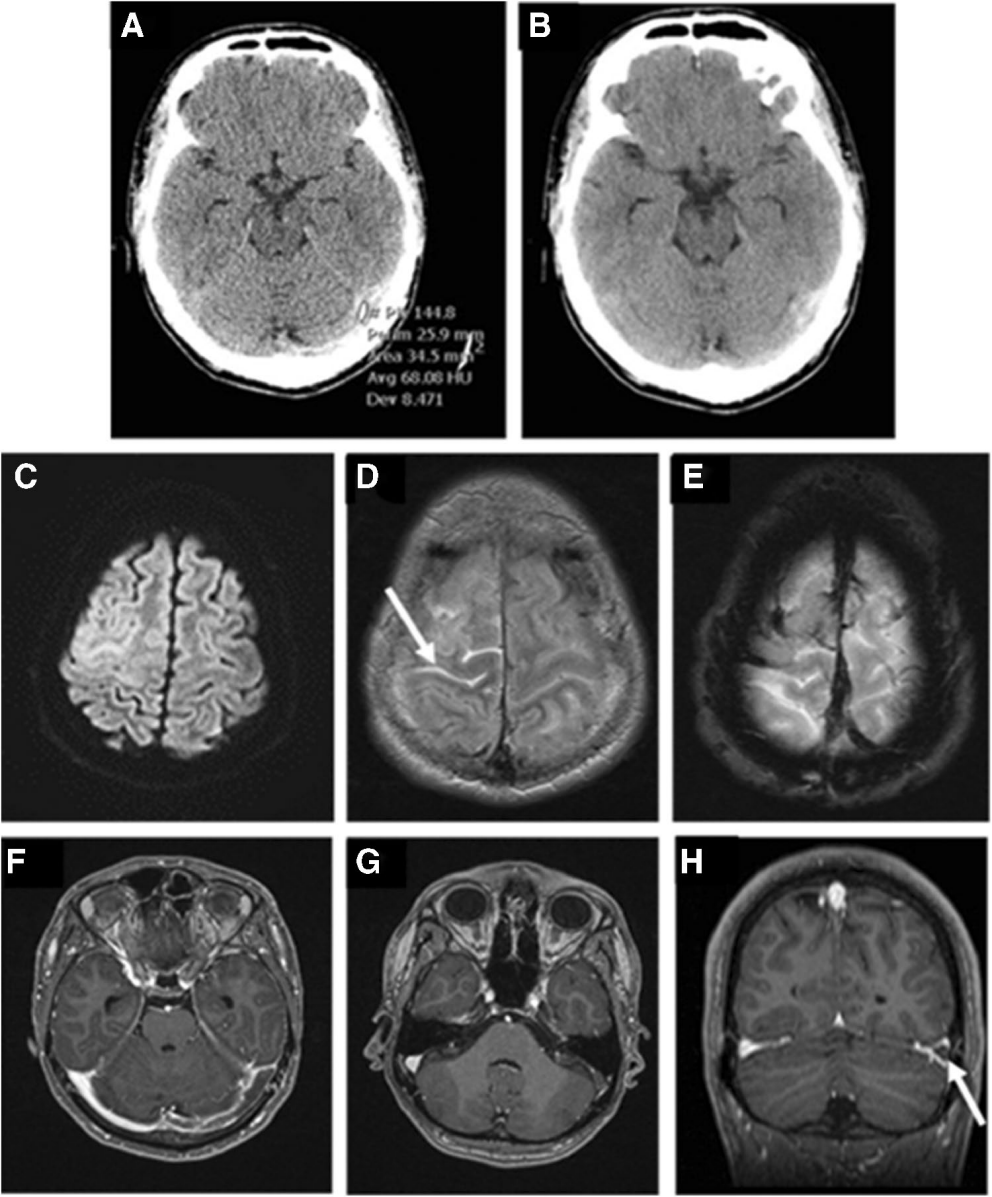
A, B Unenhanced CT scan. Cord sign of the left sigmoid sinus at an average density of 68 Hounsfield-Units. C Hyperintense sulcal diffusion restrictions of the right hemisphere. D Corresponding hyperintense SAB (arrow) in FLAIR sequence. E Emphasized bridging veins in T2*-weighted imaging. F–H CE T1-weighted images show “triangular sign” of the left sigmoid sinus (arrow)
